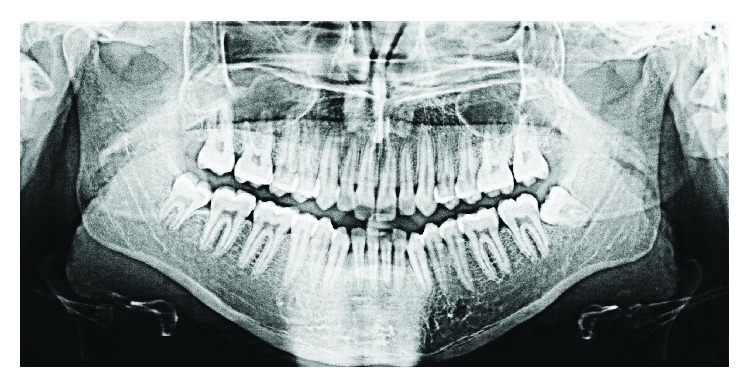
Panoramic X-ray before the excision of mucoepidermoid carcinoma.
